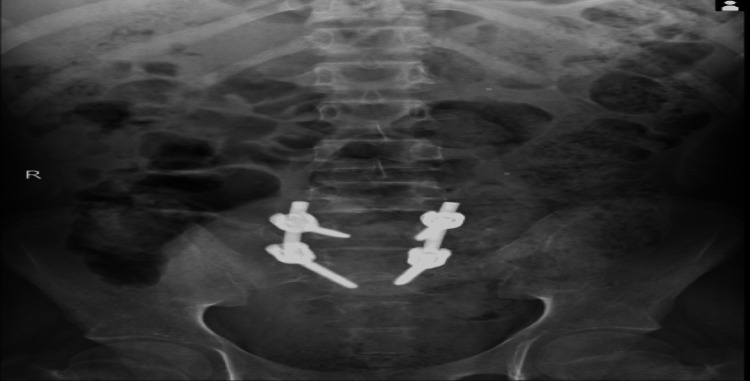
Anterior-posterior X-ray of the lumbar spine at the eighth-month follow-up demonstrating evidence of fusion
